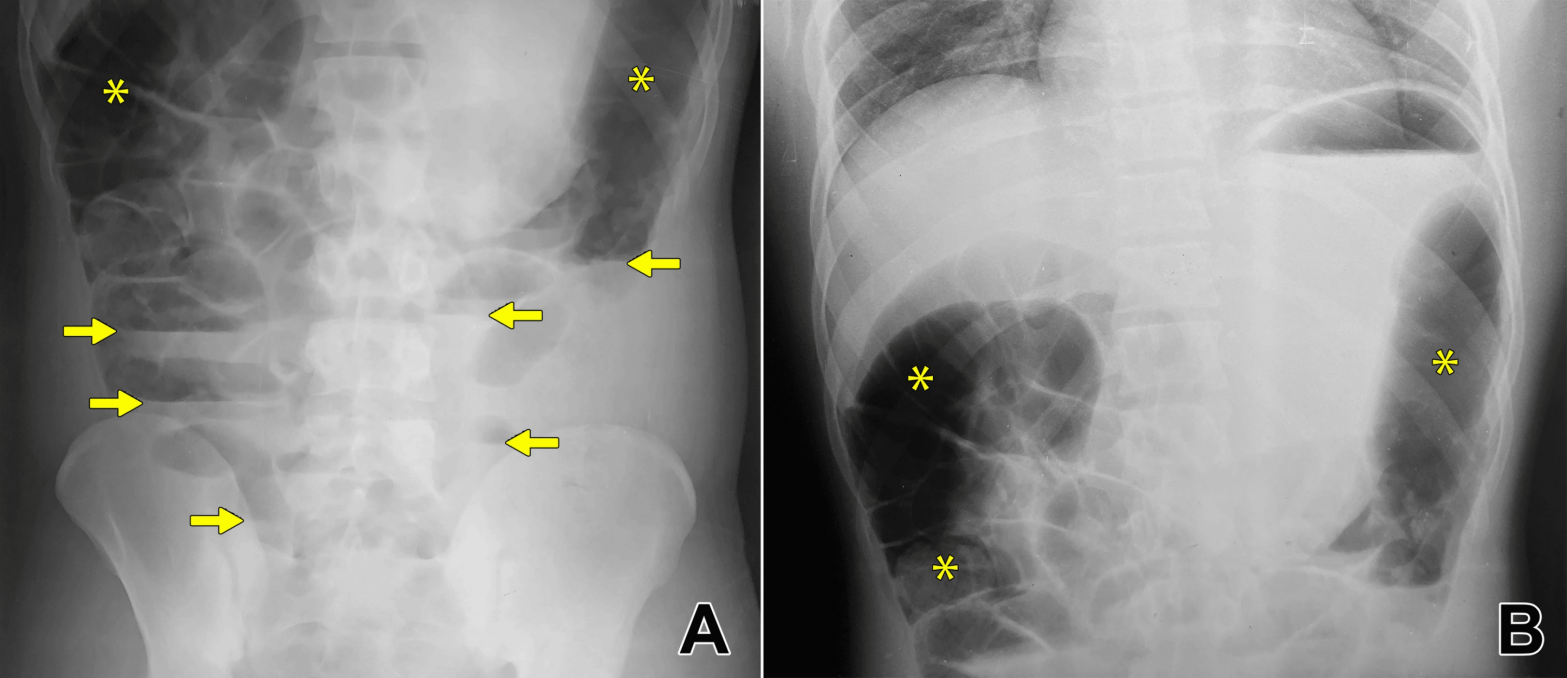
Abdominal X-rays showing dilated bowel loops with air-fluid levels. (A) Erect abdominal X-ray shows multiple dilated bowel loops (asterisk) with air-fluid levels (arrow); (B) supine abdominal X-ray demonstrates diffusely dilated bowel loops (asterisk) without a clear transition point.
